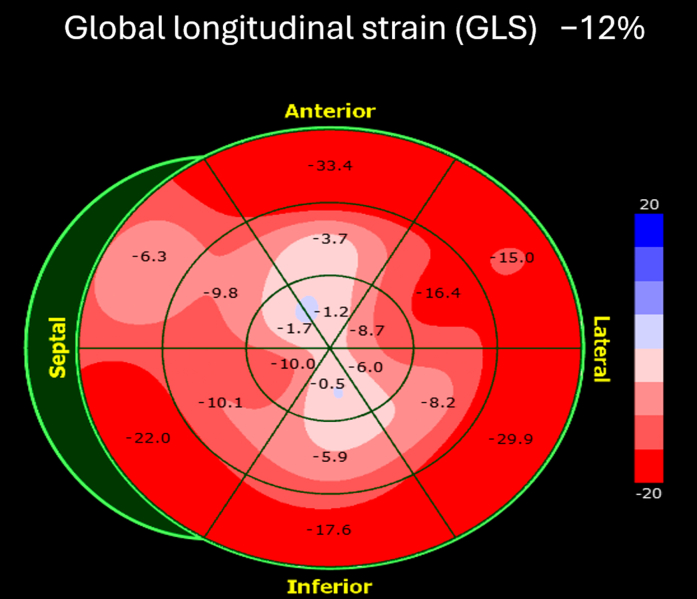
Global Longitudinal Strain Analysis by Speckle-Tracking Echocardiography Speckle-tracking echocardiography demonstrates a reduced global longitudinal strain (GLS) of −12%, with a base-to-apex gradient characterized by markedly reduced strain in the apical segments and relatively preserved basal strain.
